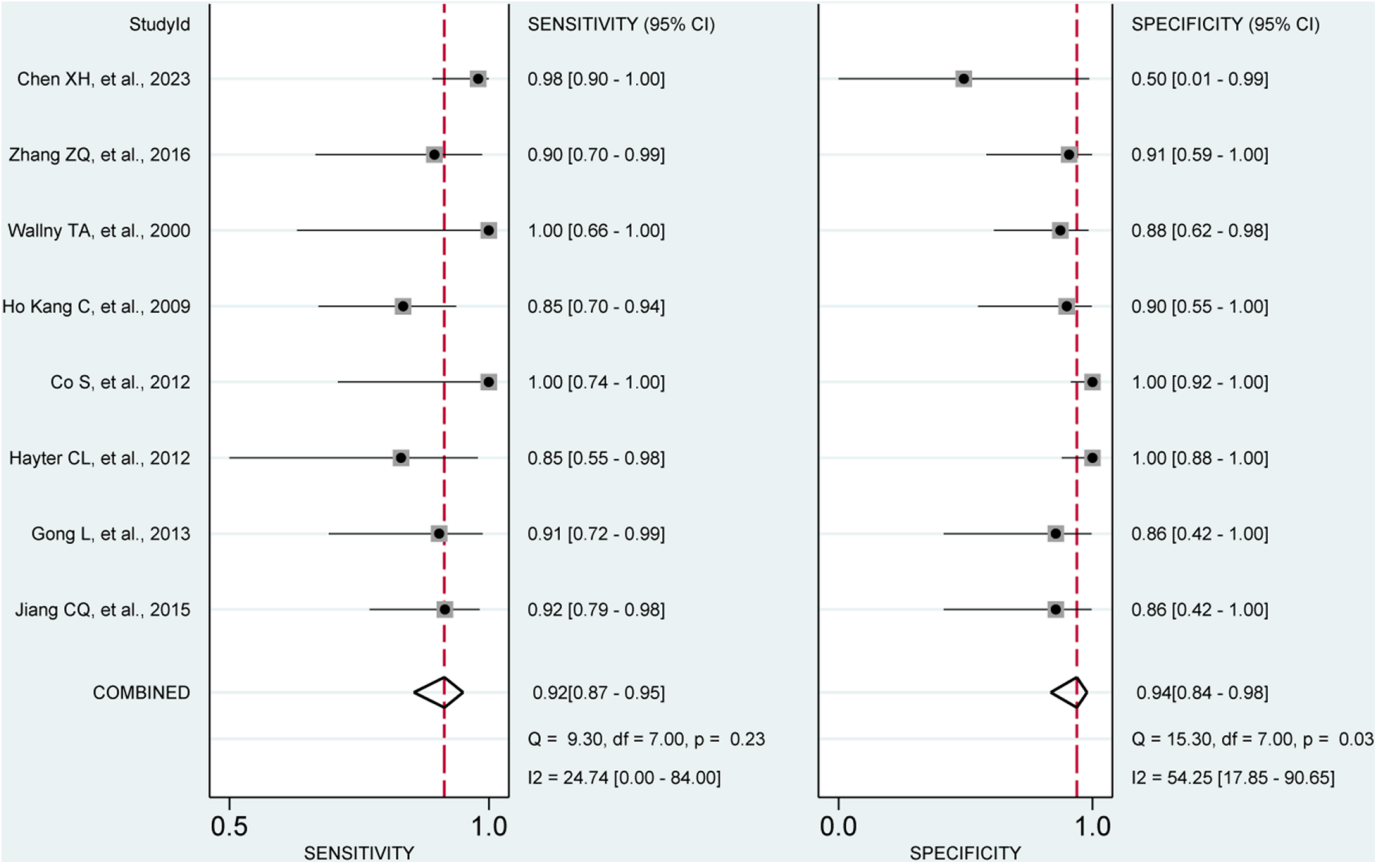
Forest plots of the pooled sensitivity and specificity to diagnose full-thickness rotator cuff tears with the corresponding 95% confidence region. Three-dimensional sonography for the diagnosis of patients with full-thickness rotator cuff tear (eight studies, the pooled sensitivity = 0.92, *I*^2 ^= 24.74%; the pooled specificity = 0.94, *I*^2 ^= 54.25%). CI, confidence interval.
